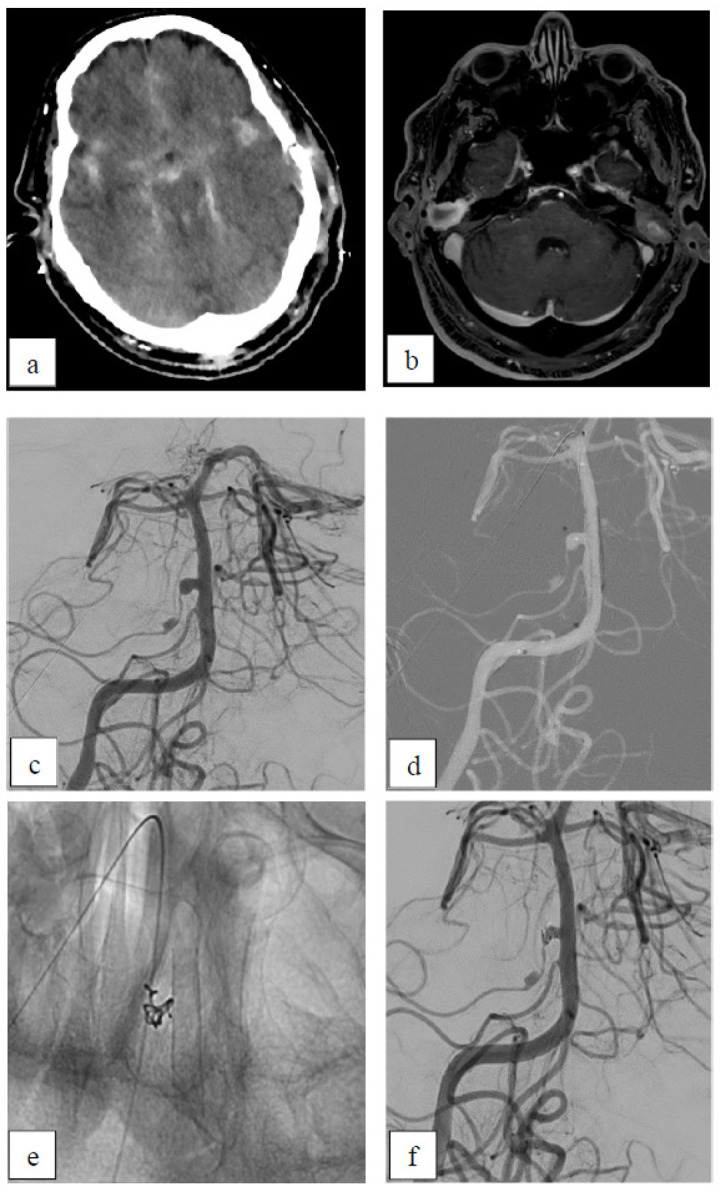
Inflammatory aneurysm. (a) Native CT scan showing SAH within the basal cisterns. (b) Postcontrast axial T1-weighted MRI showing bilateral mastoiditis. (c) Pre-interventional right VA angiogram with the two inflammatory aneurysms of the right AICA. (d) Roadmap showing the first delivered p48 HPC and the tip of the microcatheter distally located after perforation of the aneurysm. (e) X-ray after coiling of the perforated proximal AICA aneurysm and after implantation of p48 HPC into the BA. (f) Directly postinterventional DSA with subtotal filling of the proximal and total filling of the distal inflammatory aneurysm.
